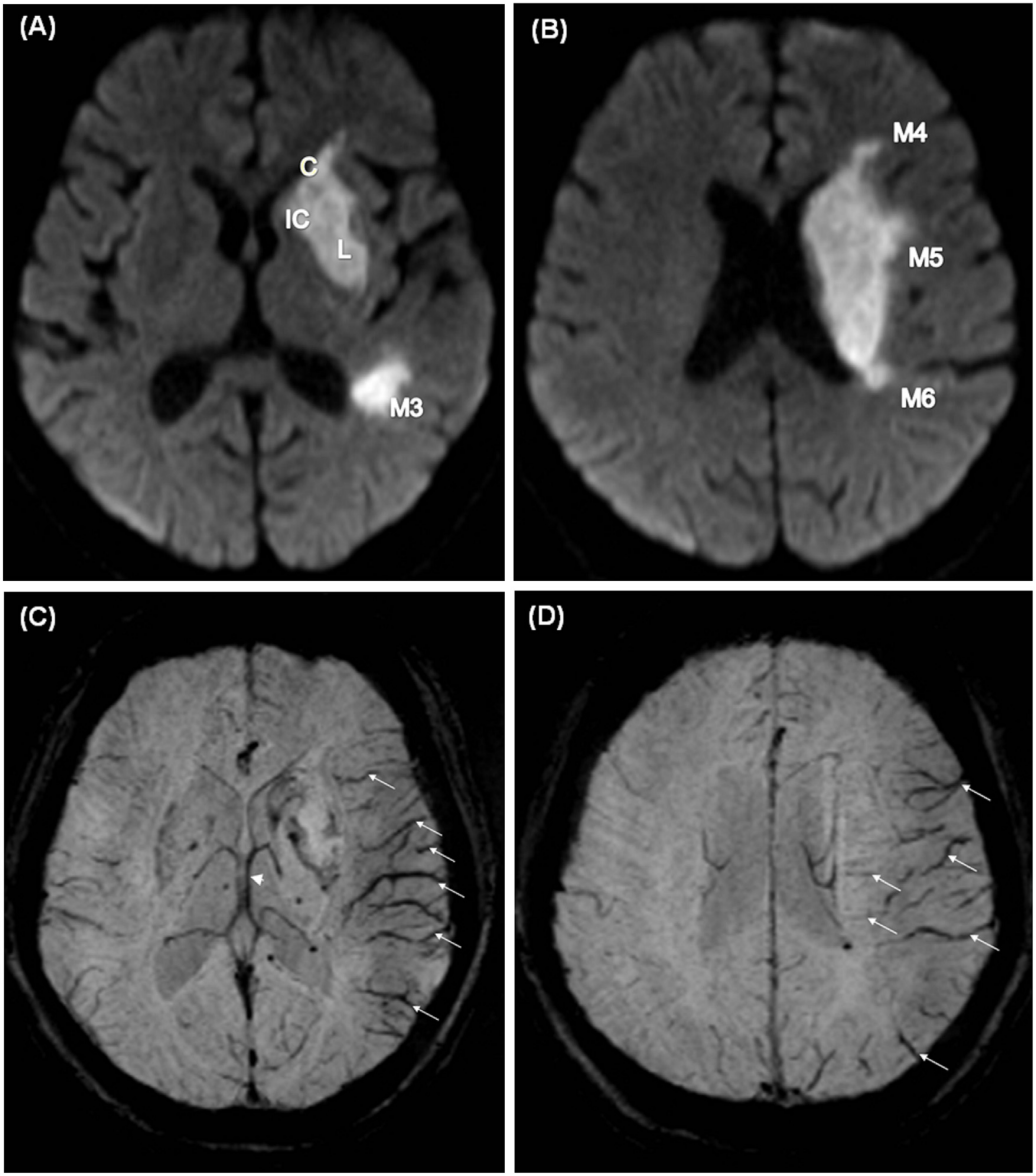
A 63-year-old woman had a diagnosis of left middle cerebral artery territory infarct. The infarct was noted in all 10 zones on diffusion-weighted or susceptibility-weighted magnetic resonance imaging with ASPECTS score = 0. Diffusion-weighted imaging (A,B) at the basal ganglion and suprabasal ganglion levels reveals the infarct in the internal capsule, lentiform nucleus, caudate nucleus, and zones M3, M4, and M5 of the middle cerebral artery. Susceptibility-weighted imaging reveals prominent hypointense cortical and medullary vessels diffusely seen in the insula and M1 to M6 zones of the left middle cerebral artery territory. Engorged deep veins and thalamostriate artery over the lesion side compared with the healthy side were also noted. Involved M1 to M6 zones and insula lost 7 points and an engorged thalamostriate vein lost 3 points. The prominent vessel sign score was 0 (10–7–3 = 0). Susceptibility-weighted imaging (C, D) at the basal ganglion and suprabasal ganglion levels reveals prominent vessel signs in the cortical veins (arrows), medullary veins (arrows) and thalamostriate vein (arrowhead).
